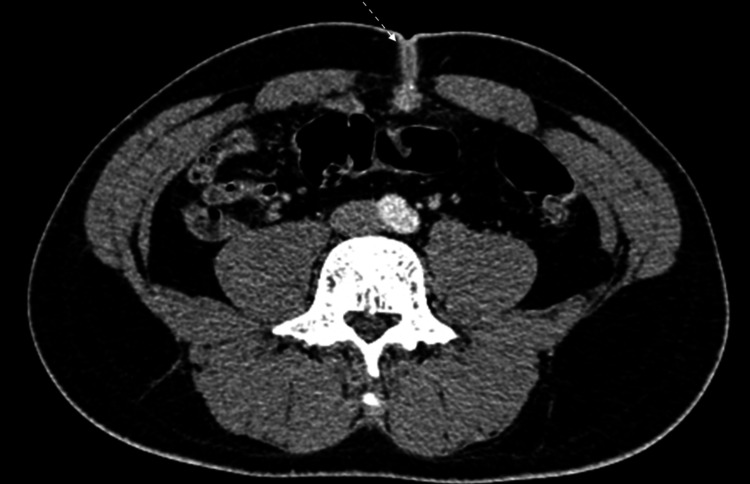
Axial image of a contrast-enhanced abdominal computed tomography showing a thickened walled peripherally enhanced collection (white dotted arrow) extending from the umbilicus towards the pre-peritoneal space.
